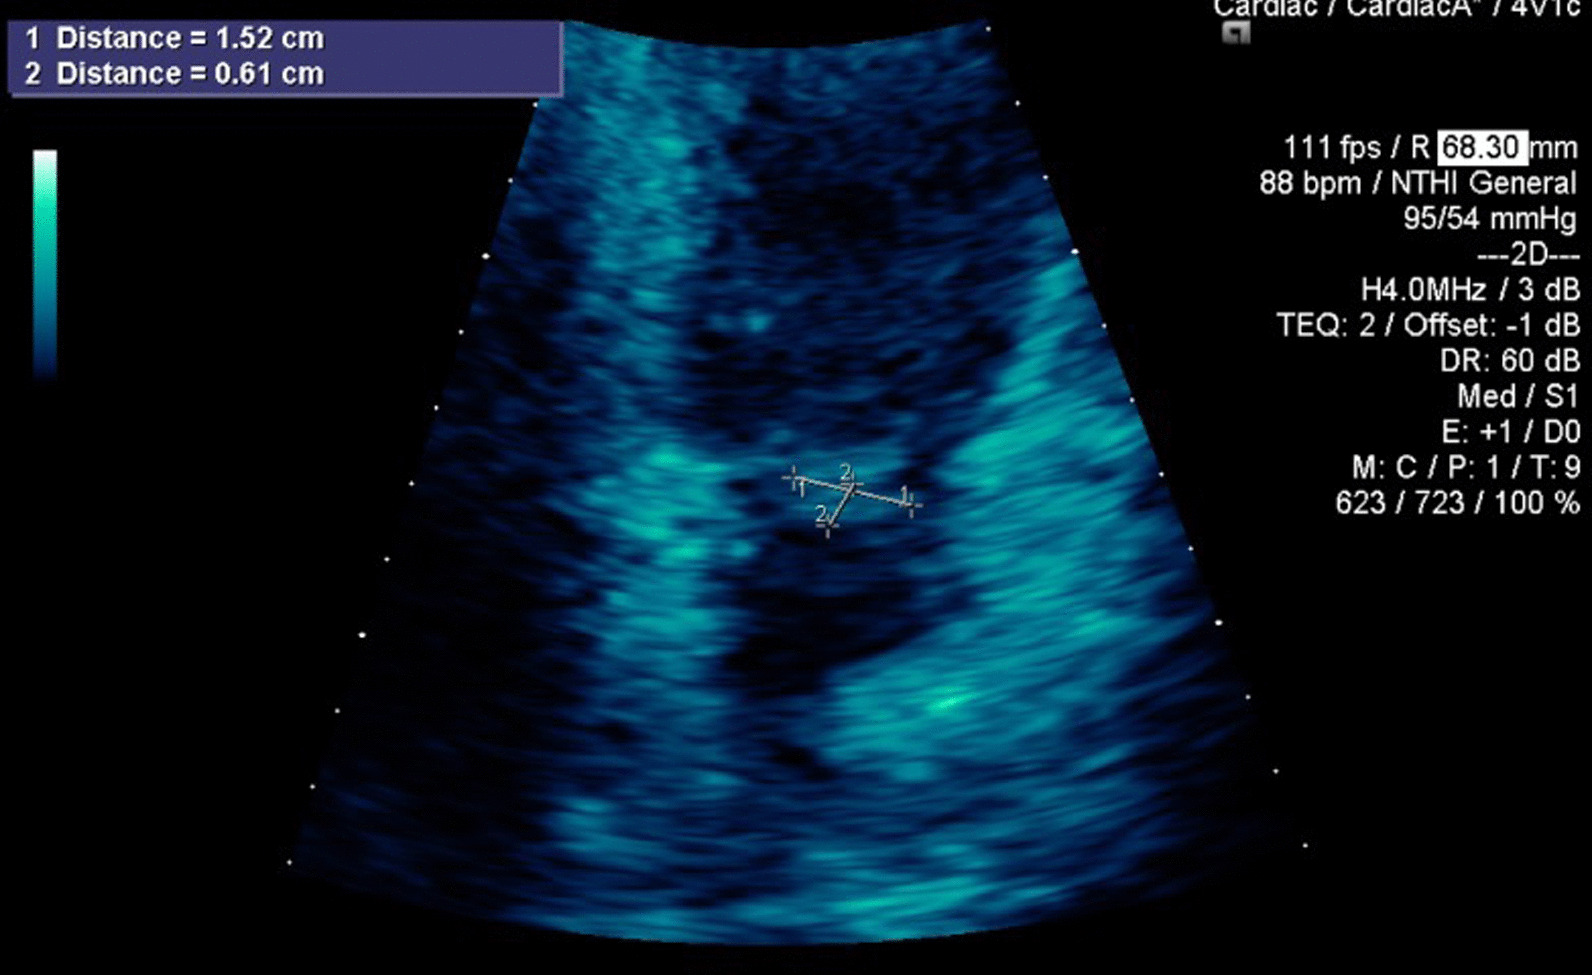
Transthoracic echocardiography revealed a 1.2 × 0.5 cm mobile echogenic density on the anterior leaflet of the mitral valve
